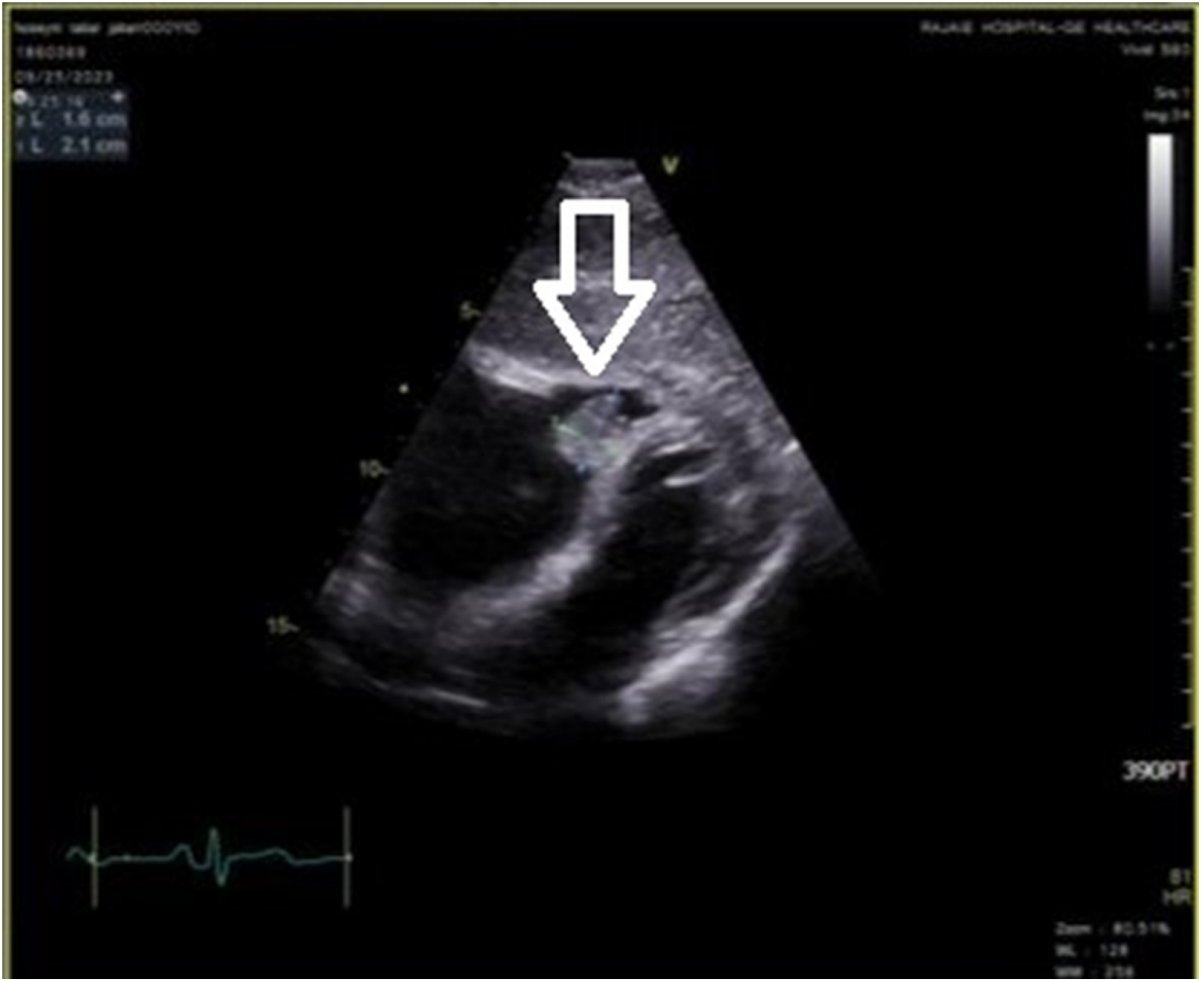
TTE of a 44‐year‐old male with pleuritic chest pain, before surgery (subcostal view). The mass is visualized in the parasternal short axis view, with the cystic component being more prominently featured (white arrow). Importantly, no evidence indicates attachment of the mass to the tricuspid valve or the right ventricular outflow tract. TTE: transthoracic echocardiography.
